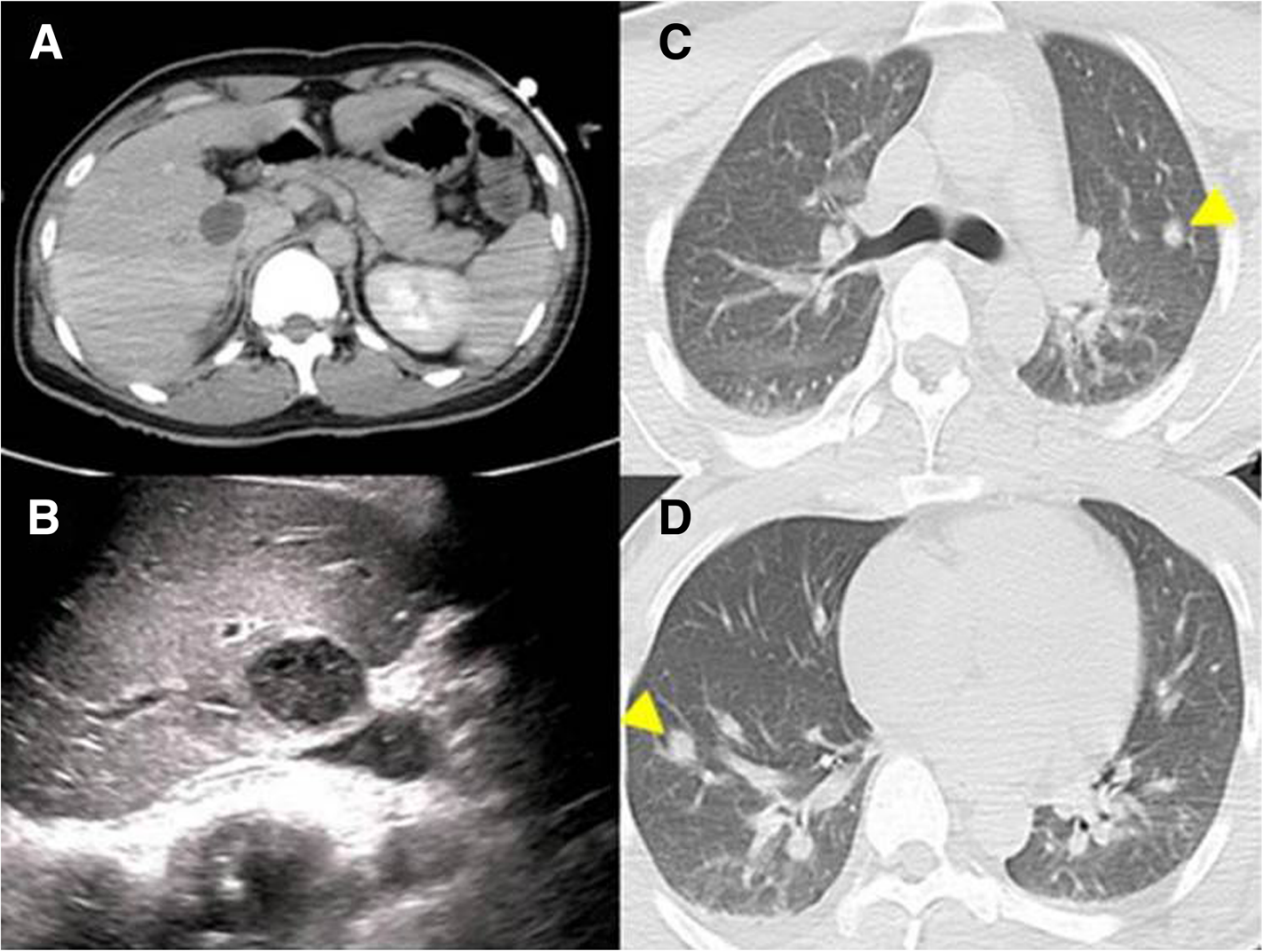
Images of computed tomography and abdominal ultrasound. A: Contrast computed tomography revealed a 3-cm low density area in segment 6 of the liver. Slight ring-shaped contrast effects were observed in the lesion area. Liver abscess was diagnosed. B: Abdominal ultrasound revealed an oval-shaped, hypoechoic lesion with clear borders in segment 6 of the liver. C, D: Multiple nodes were observed in the left superior lobe and right inferior lobe of the lung (arrowheads). Septic emboli were suspected. These nodes completely disappeared with treatment.
